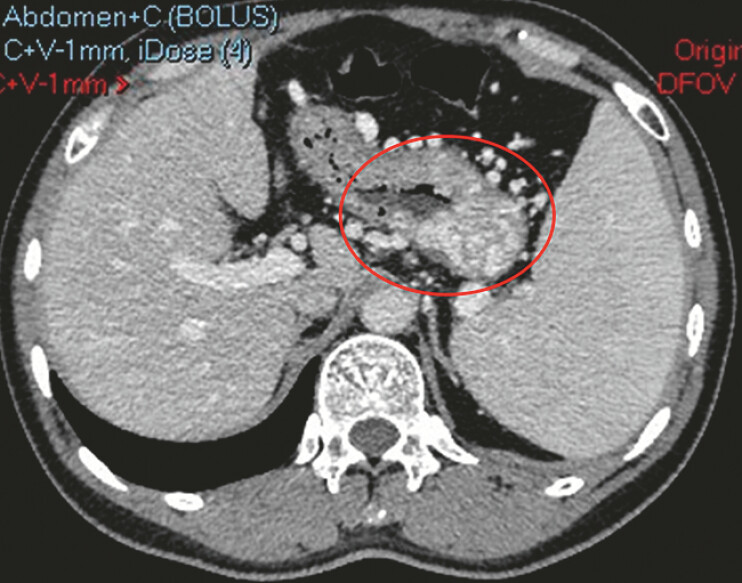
Abdominal computed tomographic angiography shows multiple dilated and tortuous vascular shadows in the stomach.
